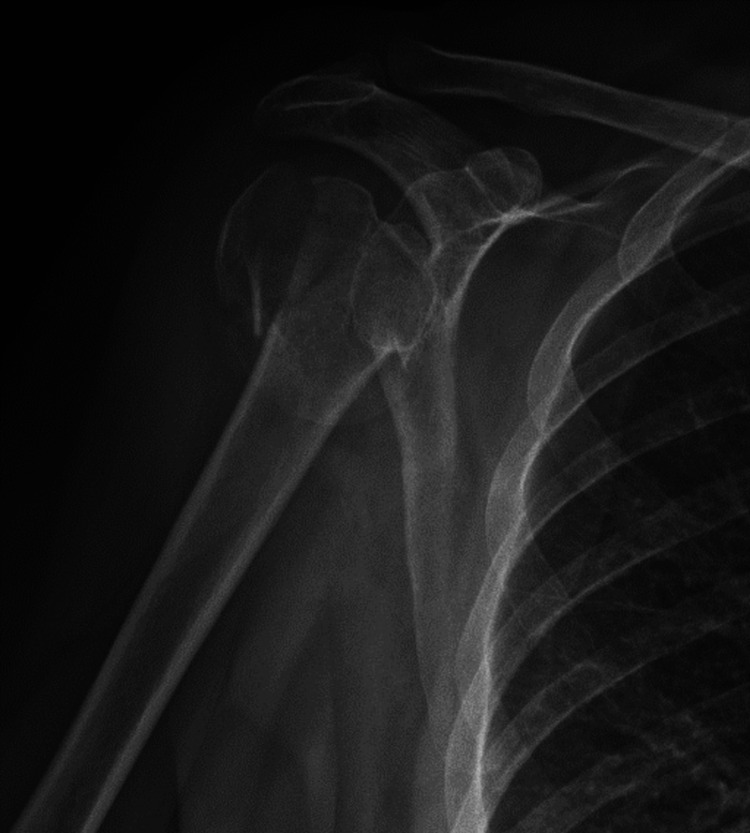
A 61-year-old female’s preoperative X-ray.
